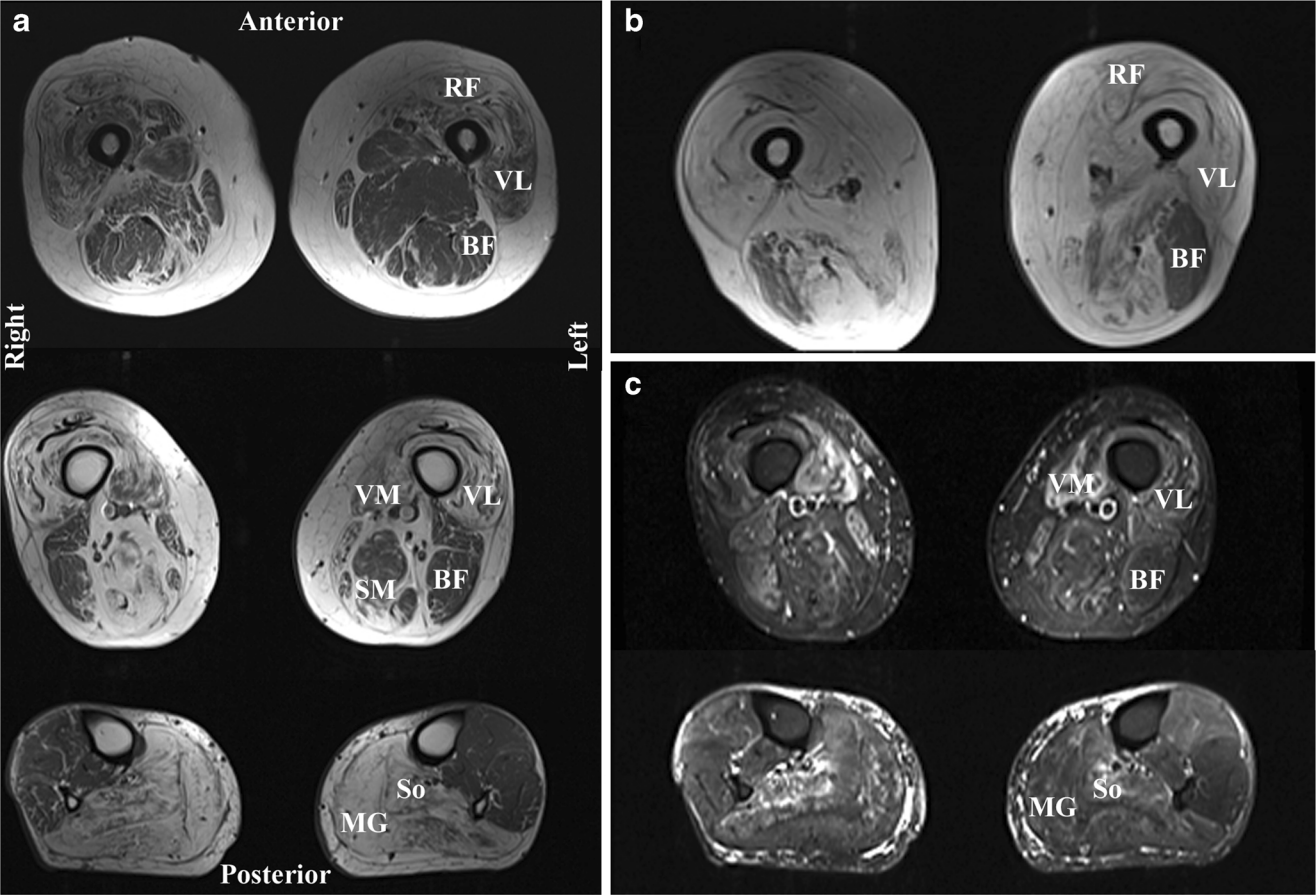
Typical MRI appearances in a patient with IBM. (a) Axial T1-weighted images of the mid-thigh (top), distal thigh (middle), and mid-calf (bottom). The thigh shows intramuscular fat accumulation, evident as hyperintensity; most notably within the quadriceps (RF: rectus femoris; VL: vastus lateralis; VM: vastus medialis), especially in the distal thigh. Hamstring involvement is asymmetric, with semimembranosus (SM) relatively spared on the left. In the calf the medial gastrocnemius (MG) is completely replaced by fat, with the soleus (So) also severely affected. (b) Axial T1-weighted image at mid-thigh of the same patient six years later shows significant progression of intramuscular fat accumulation, with only the biceps femoris (BF) relatively unaffected. (c) Axial STIR images at distal thigh (top) and mid-calf (calf) in the same patient at baseline. Acute muscle inflammation is evident as hyperintensity, most markedly in the vastus medialis and soleus
